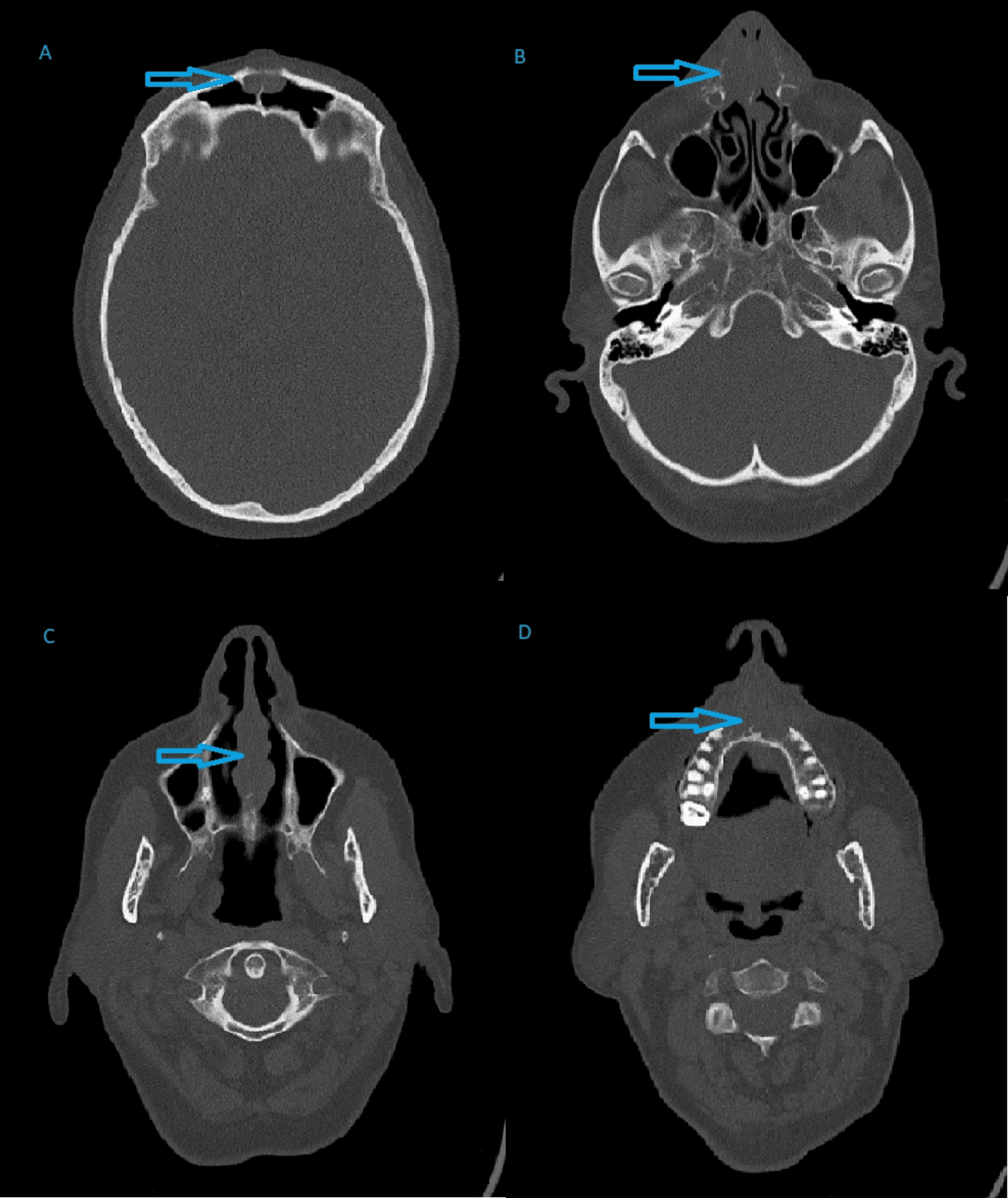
CT Scan in Sinonasal Sarcoidosis Case CT scan showing (A) a central frontal soft tissue lesion eroding the anterior wall of the frontal sinuses and extending to the adjacent subcutaneous tissue. (B) Further soft tissue thickening and bony erosions in the bridge of the nose and nasal bone with involvement of the distal nasolacrimal ducts and extension to the nasolabial folds. (C) Lobulated soft tissue mass involving the nasal septum inferiorly. (D) Erosion of the alveolar process of the maxillary bone with extension to the hard palate.
